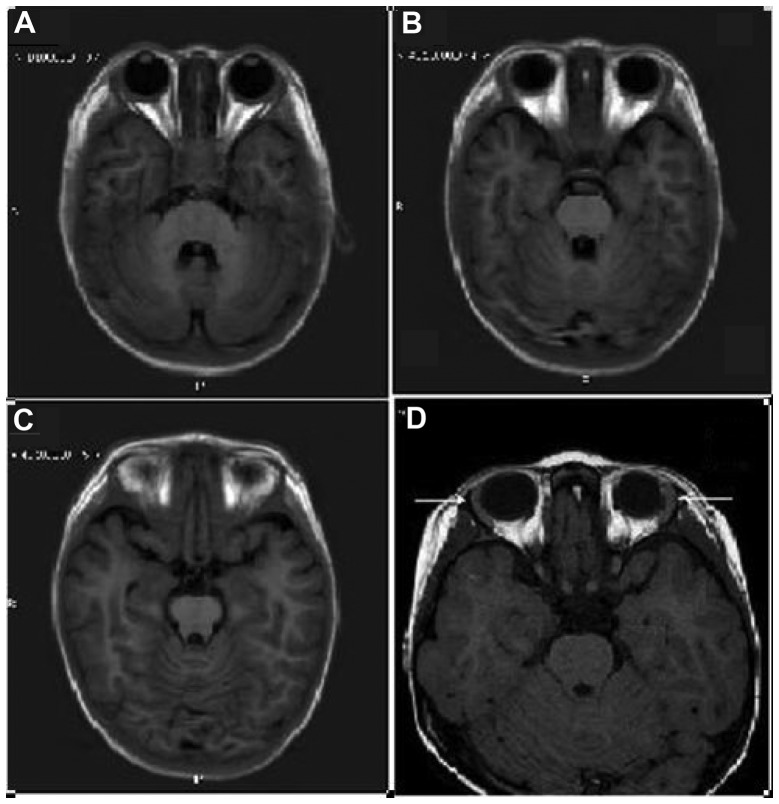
Lacrimal MR images of patient 2 compared with an image of a healthy child. (A-C) Axial T1-weighted, non-contrast MR images of patient 2, showing bilateral lacrimal gland agenesis. (D) Lacrimal gland MR image of a healthy child. An isointense internal signal is shown on the T1-weighted images (arrows indicate the lacrimal glands). MR, magnetic resonance.
